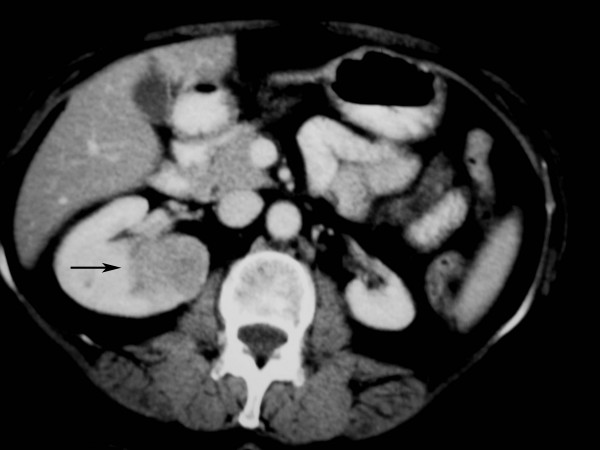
Contrast enhanced CT scan shows a right kidney mass suggestive of primary RCC measuring 3.2 × 3.2 × 3.8 cm with a central portion adjacent to the renal pelvis (black arrow).
